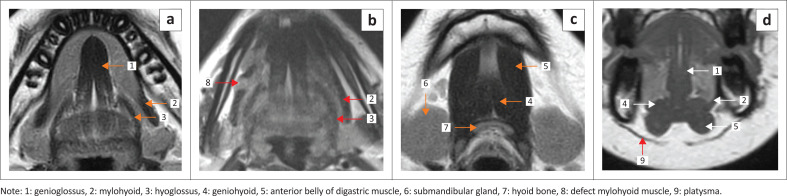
MRI anatomy of the floor of the mouth on T1 weighted (T1W) images indicating the muscles of the floor of the mouth and the extrinsic muscles of the tongue. Inferior axial (a), mid axial (b), superior axial (c) and coronal (d).
